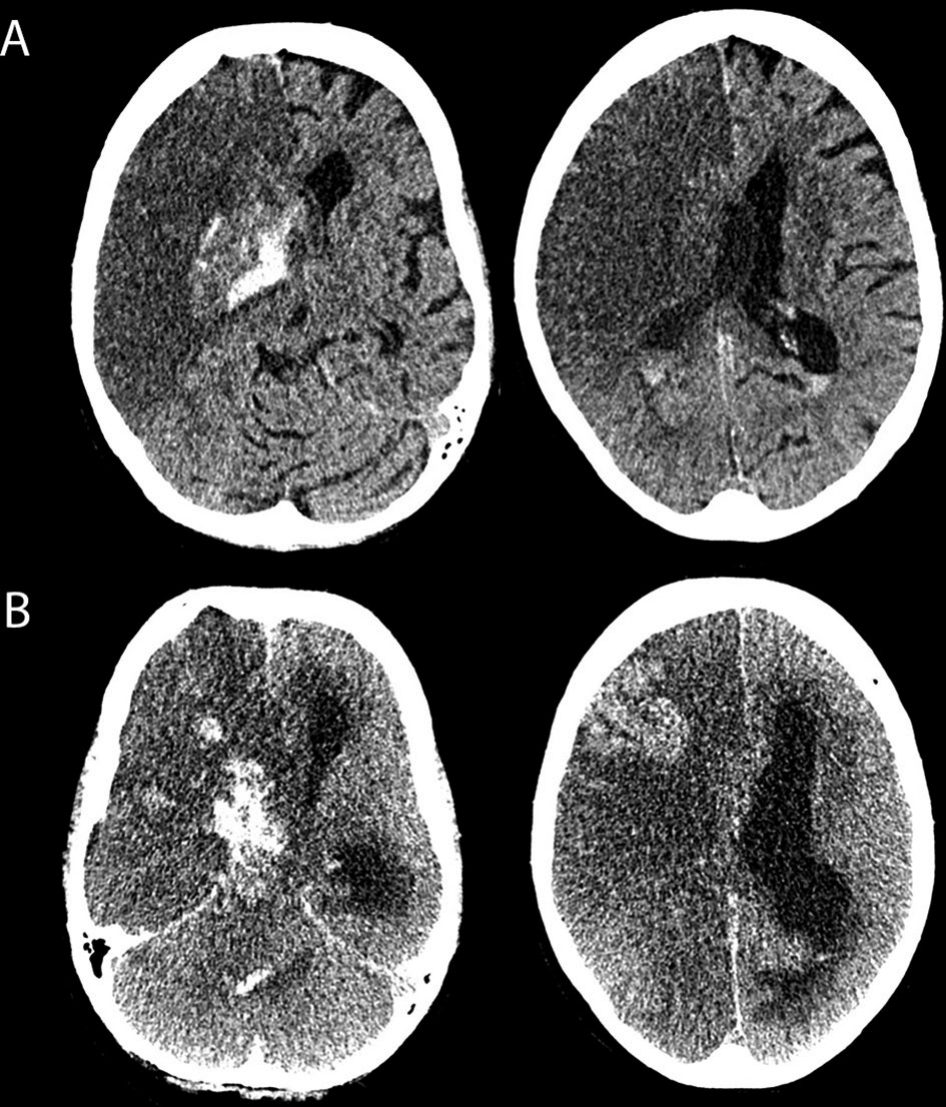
Patient with large right-sided hemispheric infarction that resulted in cerebral edema, secondary intracerebral hemorrhage, intraventricular hemorrhage, hydrocephalus and transtentorial herniation. The patient was screened positive by DETECT on day 4 (6 a.m.) following admission to neurocritical care. A Early follow-up CT on day 2 used for independent neuroimaging assessment for the potential of developing brain death. B CT scan on day 5 when brain death was confirmed
